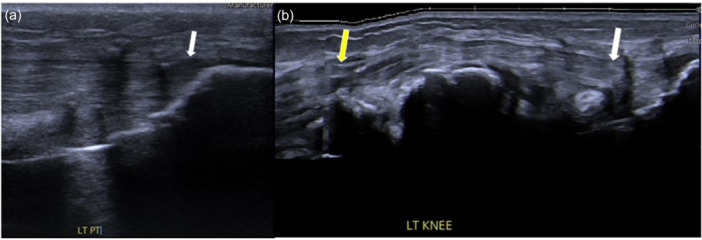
Panoramic ultrasound performed 6 months after surgery demonstrating intact graft with ligamentization extending from (a) anteromedial aspect of quadriceps tendon in the distal portion of the thigh, extending over the patellar tendon and (b) reaching distally up to the tibial tuberosity.
